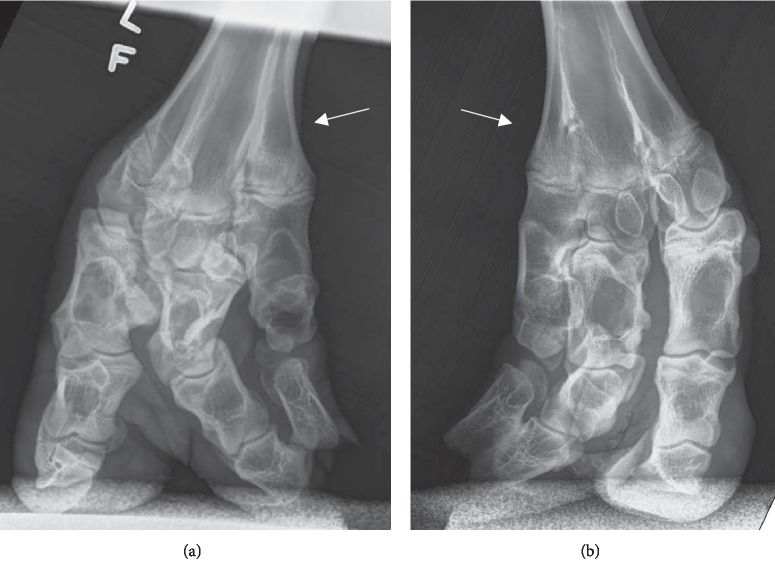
Palmodorsal radiographs of the front feet of Dexter heifer Case 2 at 11 mo of age. (a) Left front foot. (b) Right front foot. Both feet presented segmented bone development indicating an extra digit next to each medial digit. Each foot presented an extra fused section of the metacarpus (shown by the arrows). The extra digits extended the length of the foot with apparent fusion with the third phalanx of the normal digits.
